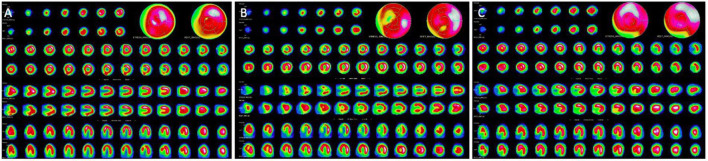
Overview of nuclear imaging in pediatric cardiology. CT, computed tomography; PET, positron emission tomography; SPECT, single-photon emission computed tomography.
